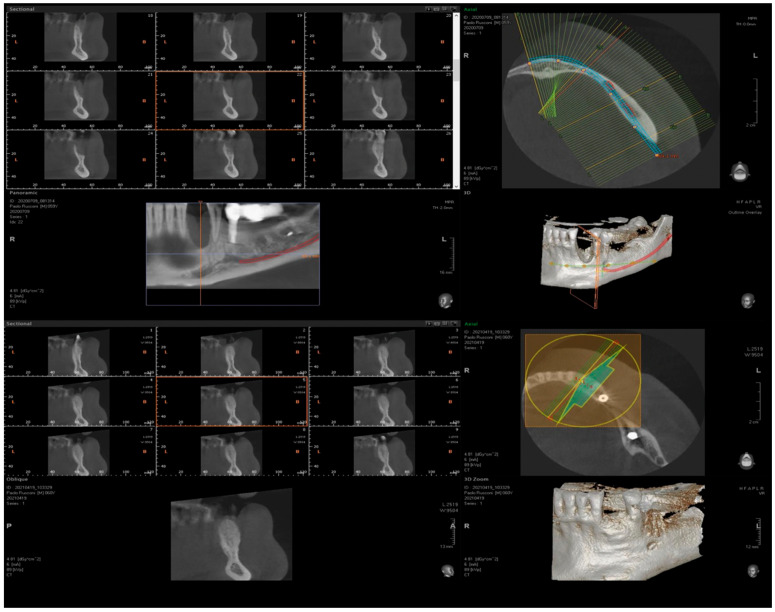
Comparison of CBCT at baseline and 8 months after GBR.
